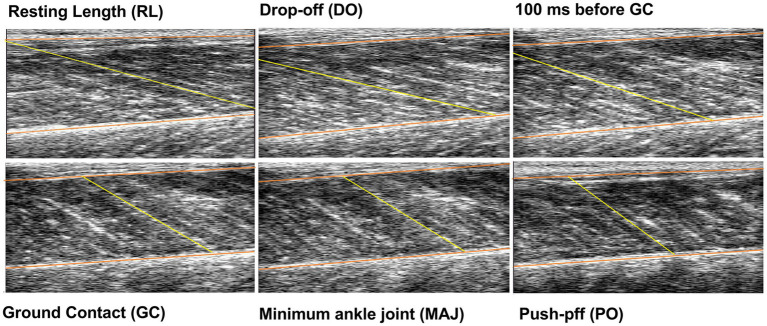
Ultrasound images extrapolated from the video recorded during a drop jump (DJ) from a representative subject. The chosen jump time points are represented: resting length (RL; the participant being bipedally standing on the platform with 0° of ankle and knee flexion), drop-off (DO; 12 ms before starting to fall), 100 ms before ground contact (GC; during pre-activation), GC (beginning of the braking phase), minimum ankle joint angle (MAJ; end of the braking phase), and push-off (PO; representing the last frame before the ground is left and the flight phase is entered). One of the three analyzed fascicles and the superficial and deep aponeuroses have been superimposed to the images in yellow and orange, respectively.
